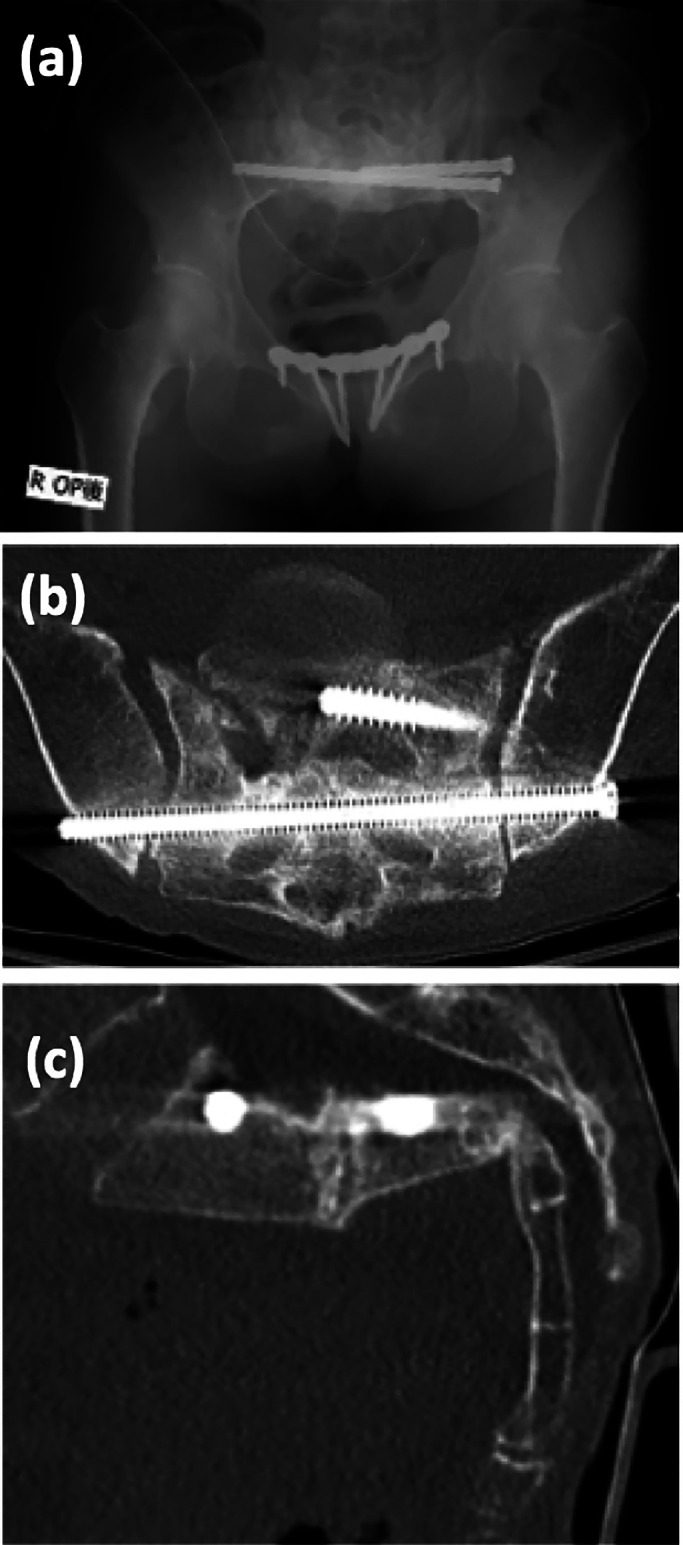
Postoperative X-ray (a), CT axial plane (b) and sagittal plane (c).
